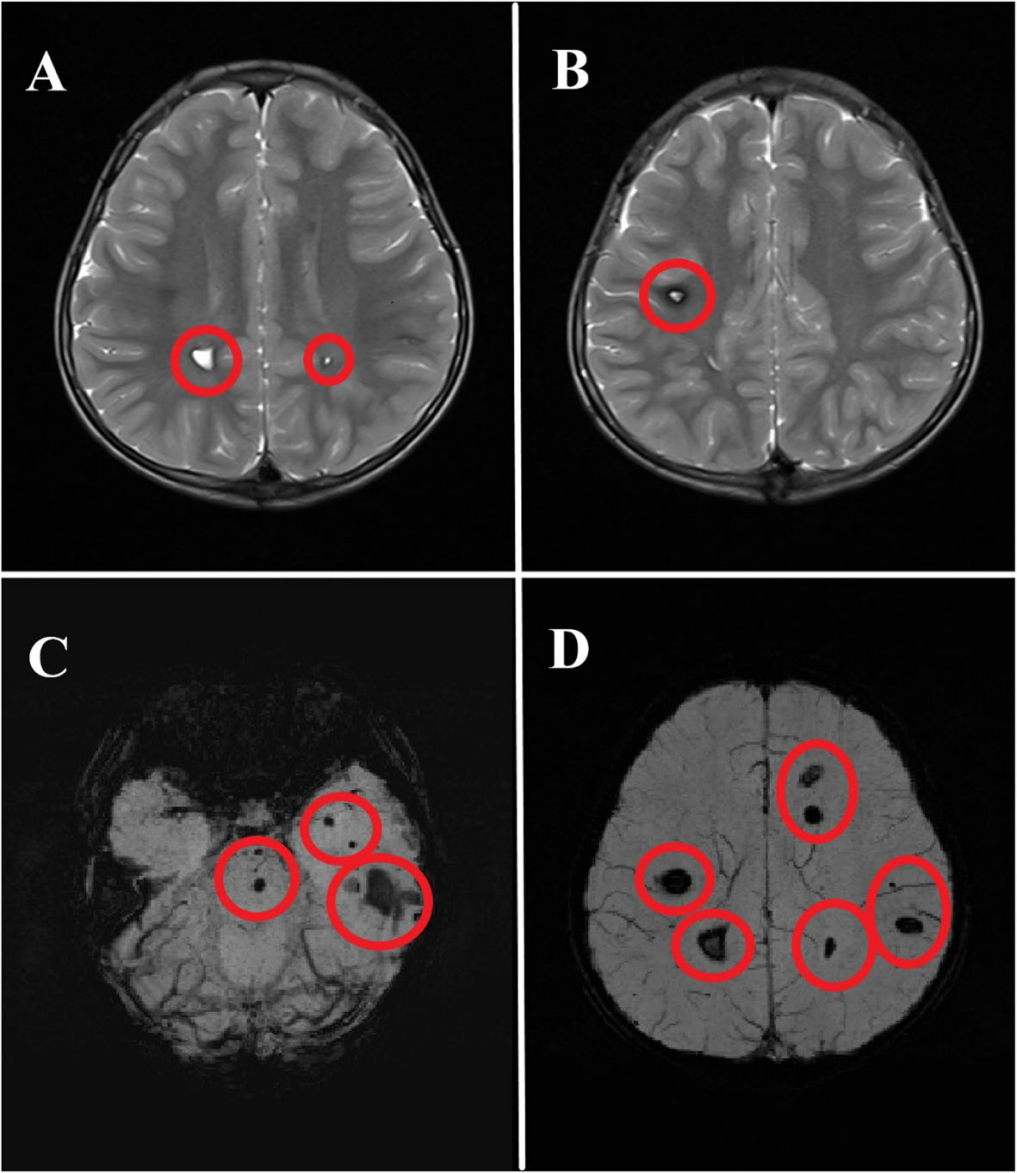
The abnormal magnetic resonance imaging (MRI) of head. a T2WI showed high signal intensity in bilateral frontal-parietal lobes (marked with red circles). b T2WI showed high signal intensity in the right frontal-parietal lobe (marked with a red circle). c SWI showed low signal intensity in multiple sites of the brain stem and bilateral temporal lobes (marked with red circles). d SWI showed low signal intensity in multiple sites of bilateral frontal-parietal lobes (marked with red circles)
